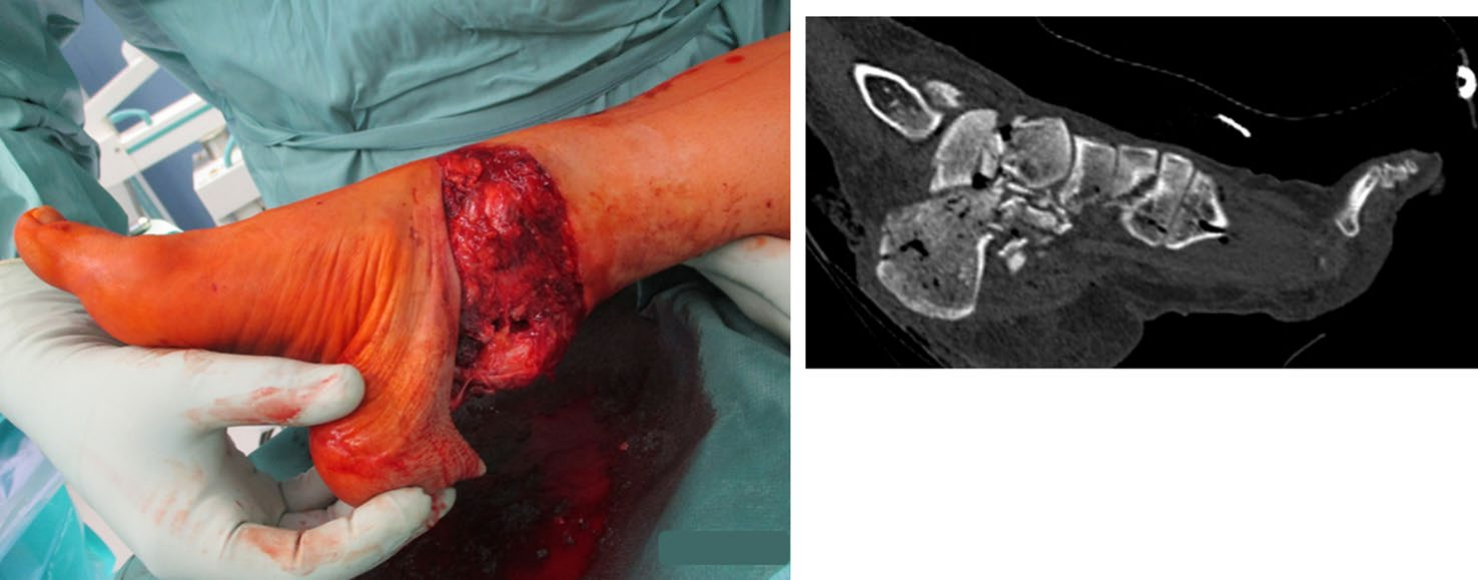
a Clinical findings of the complete degloving of the heel pad. b The initial CT scan obtained in the emergency department revealed a displaced intra-articular fracture of the calcaneus, displaced fractures of the talar neck and lateral talar process, non-displaced fractures of the navicular, first cuneiform and first metatarsal base and a subluxation at the mid-tarsal joint
